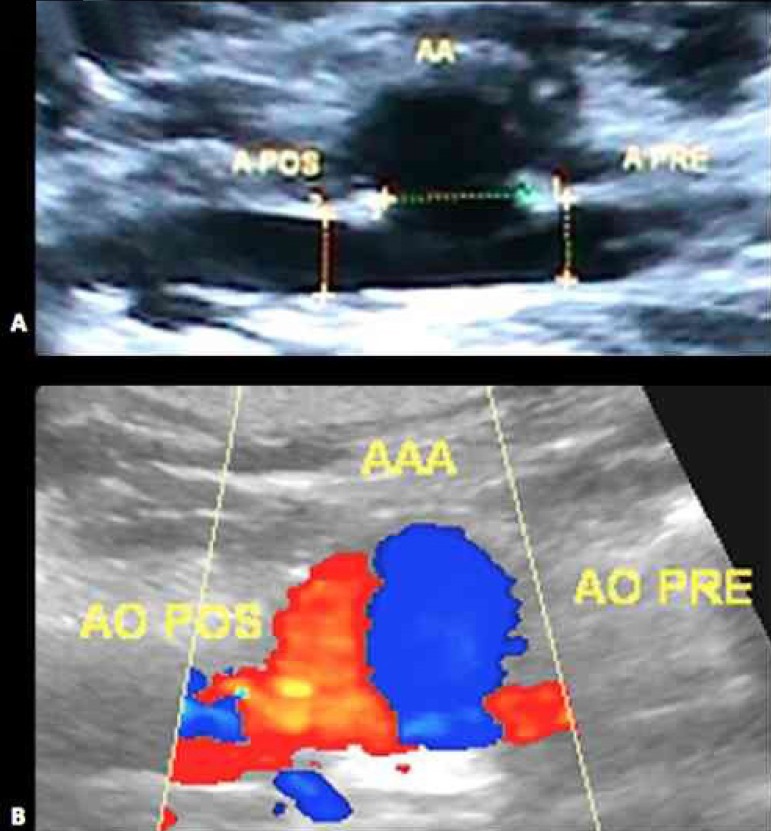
Ultrasonography B mode identifying the aneurysm (AA) and the proximal
